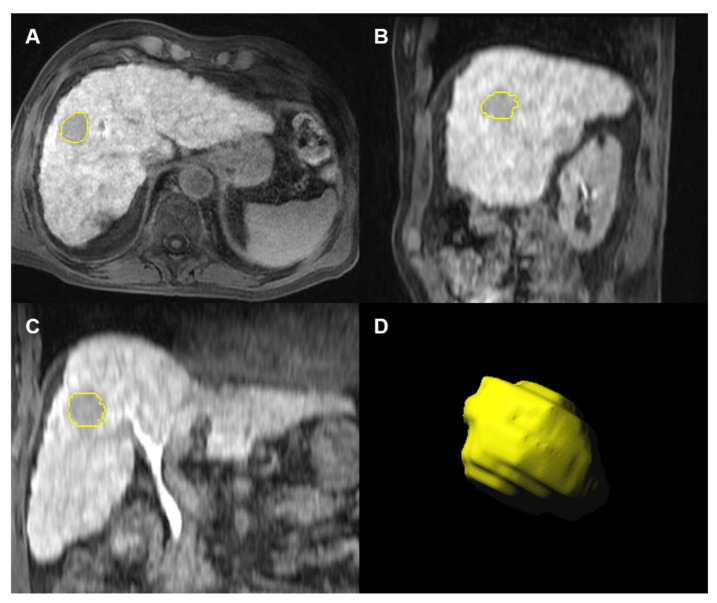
Gadoxetate disodium-enhanced MRI before transarterial embolization in a 74-year-old man with hepatitis C-related cirrhosis and a 27-mm hepatocellular carcinoma. Images shows example of whole tumor segmentation (circles) on axial (A), sagittal (B), and coronal (C) hepatobiliary phase images with volumetric lesion reconstruction (D).
